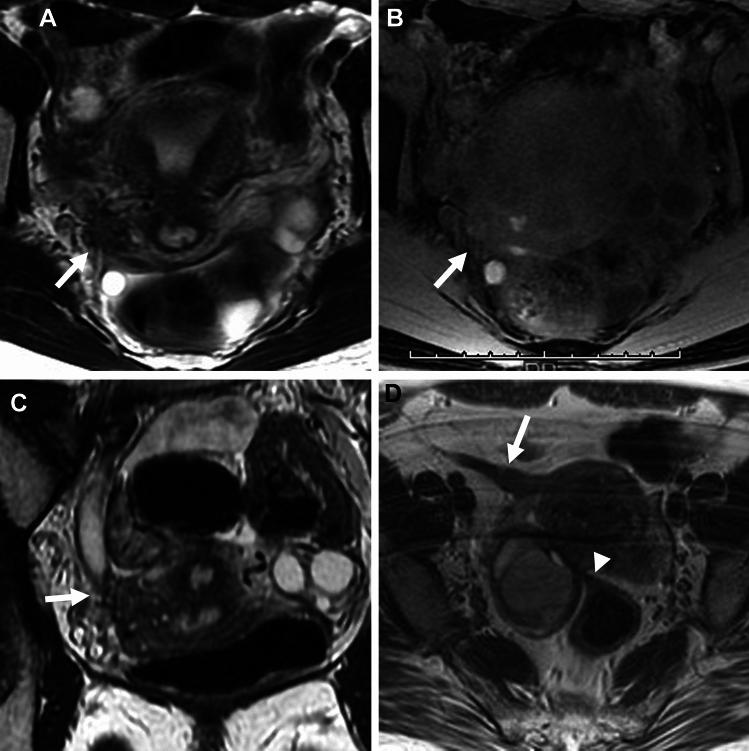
Deep endometriosis (rectosigmoid/round ligaments). A 29-year-old with a history of dysmenorrhea. A T2-weighted image and B fat-saturated T1-weighted image reveal a fibrotic plaque (arrow) on the right posterior serosal surface of the uterus to the right uterosacral ligament. Mottled T1-high signal intensity hemorrhagic foci are observed. C The right ureter is involved (arrow) resulting in right hydronephrosis on coronal T2-weighted image. A 46-year-old with right endometrioma. D On T2-weighted image, the right thickened round ligament (arrow) and a tethered appearance of the rectum to the uterus with low signal intensity faint fibrous strands (arrowhead) are revealed
